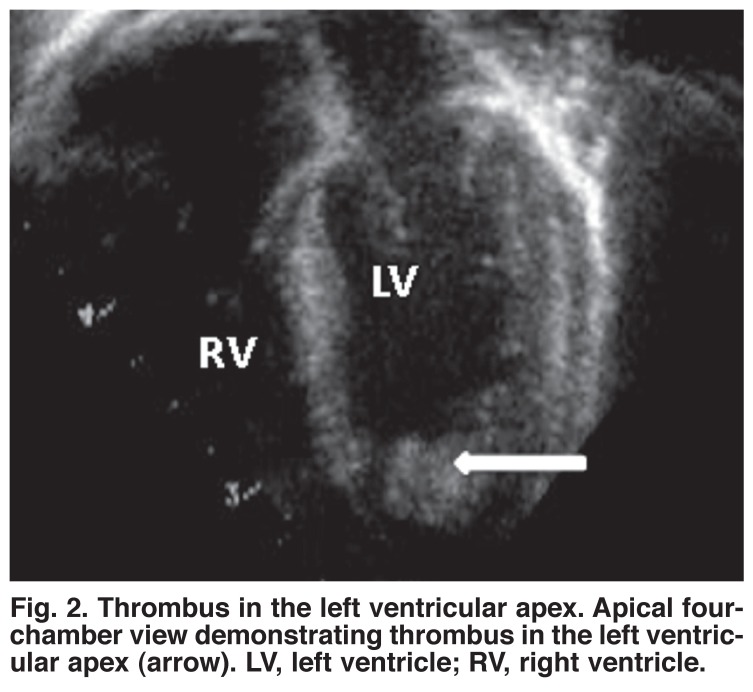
Thrombus in the left ventricular apex. Apical fourchamber view demonstrating thrombus in the left ventricular apex (arrow). LV, left ventricle; RV , right ventricle.
